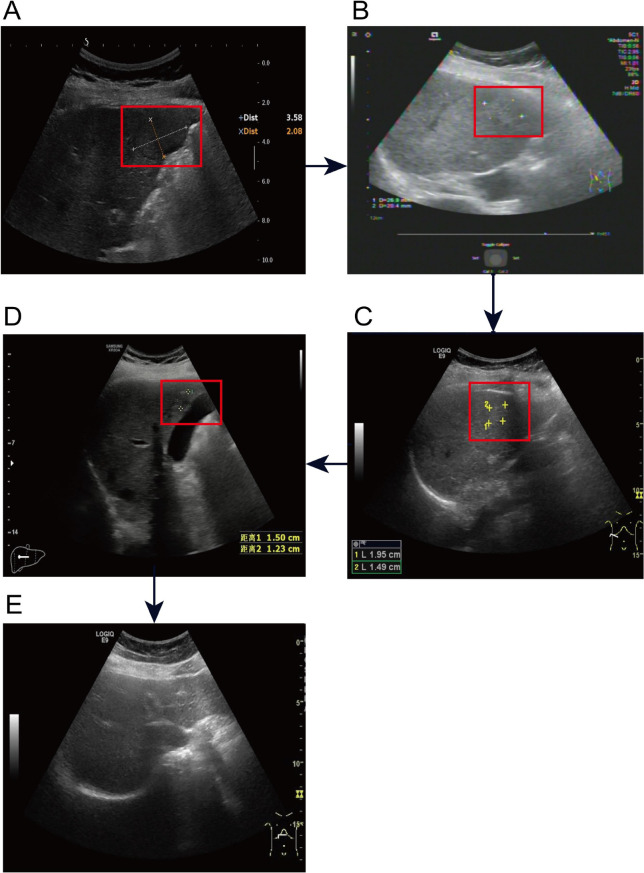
Serial liver ultrasonography demonstrating progressive regression of hepatic metastasis to complete resolution. (A) Baseline, pre-TAE (May 2, 2021): Hypoechoic nodule (3.58 × 2.08 cm) in hepatic segment V near the gallbladder fossa (red box). (B) Approximately 3 weeks after first TAE (June 4, 2021): Lesion decreased to approximately 2.7 × 2.0 cm (red box), indicating early treatment response. (C) Approximately 5 weeks after second TAE (August 5, 2021): Further regression to 1.95 × 1.49 cm (red box). (D) Approximately 8 months after second TAE (February 10, 2022): Continued regression to 1.50 × 1.23 cm (red box). (E) Approximately 19 months after second TAE (February15, 2023): No focal hepatic lesion detectable, consistent with complete radiologic regression. Arrows indicate the chronological sequence of imaging follow-up.
